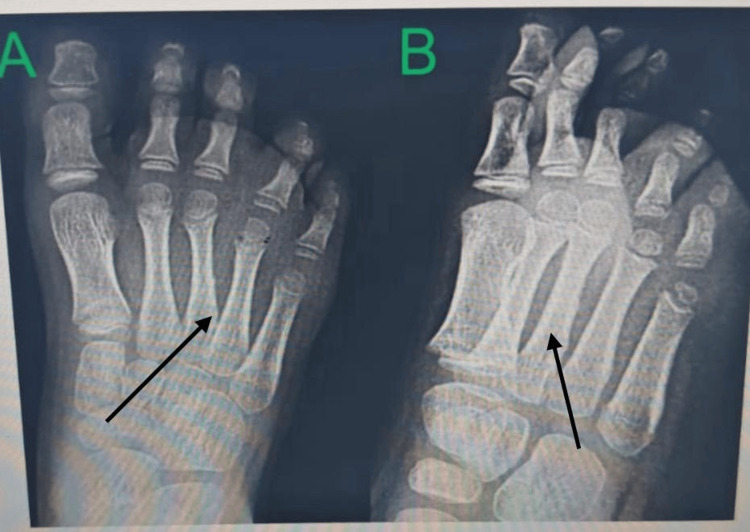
Post-operative radiograph at the six-month follow-up of foot (A: anteroposterior and B: oblique view), showing complete healing of the third metatarsal with resolution of osteolytic changes.
